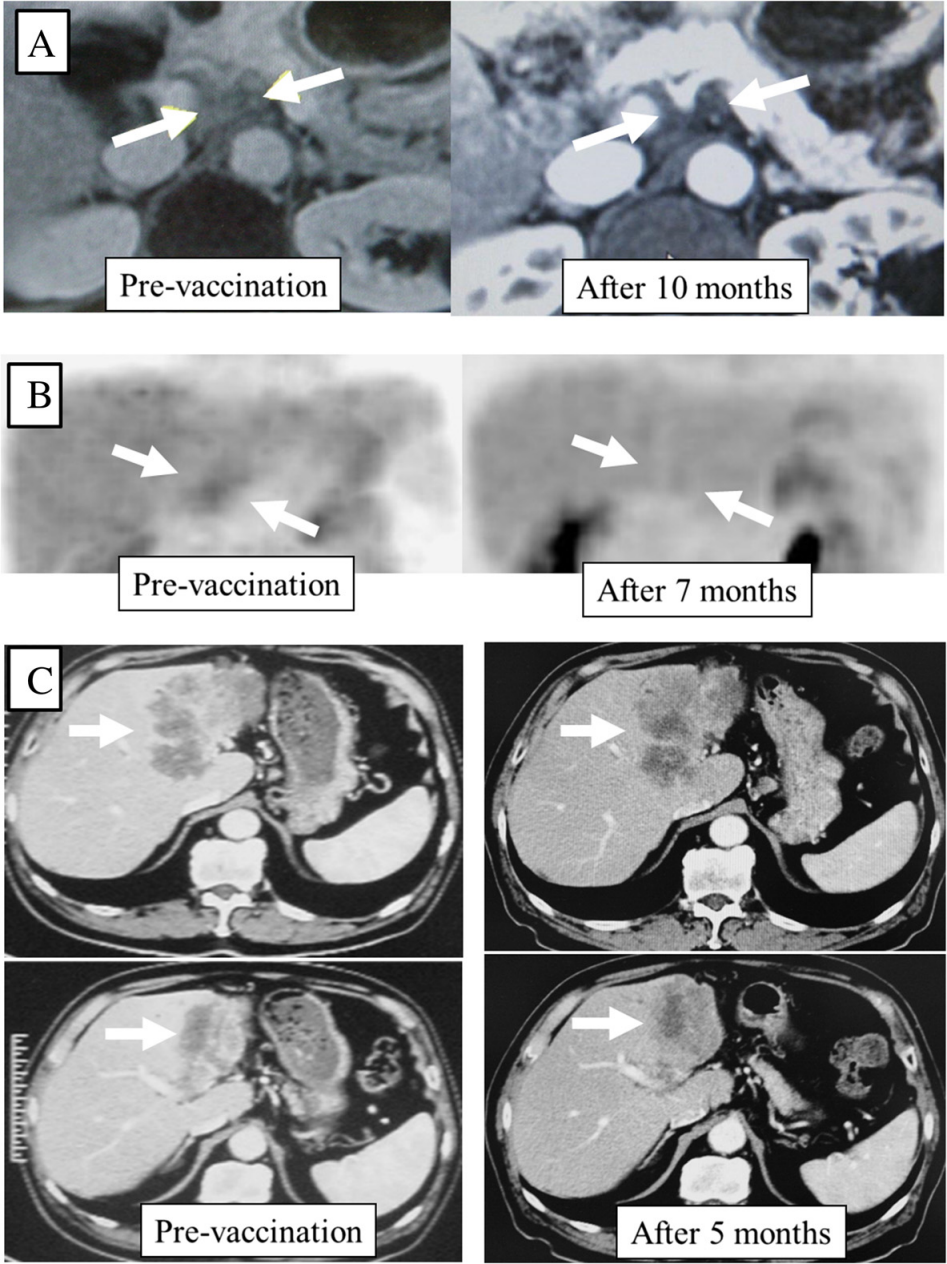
Diagnostic images of case 1 and case 3. (A) T1-weighted magnetic resonance imaging (MRI) of representative radiologic response to vaccination in case 1 who achieved complete response. (B) Positron Emission Tomography (PET) of case 1. Diffusely infiltrated lymph node metastases around the hepato-duodenal ligament by MRI (A, left side) as well as PET (B, left side) were confirmed their disappearance by MRI and PET analysis after 10 months of vaccination (A &B, right side). (C) Computed tomography in case 3 who achieved stable disease. The massive liver metastases were kept stably for 5 months.
